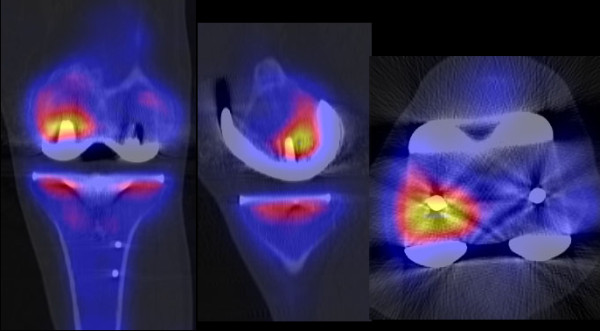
SPECT/CT imaging of a patient with mechanical loosening of femoral component as cause of painful total knee arthroplasty. (Reprint permission from Journal KSSTA, Springer).
